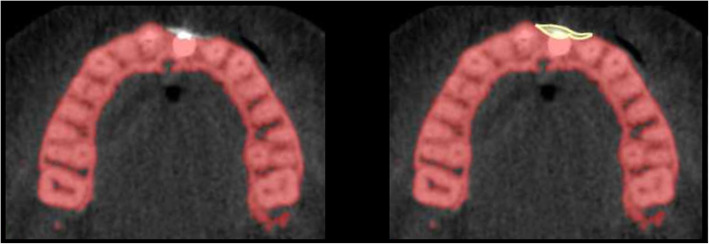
CBCT scan (axial view) of lower jaw at time point T2, 12 months after onlay graft
